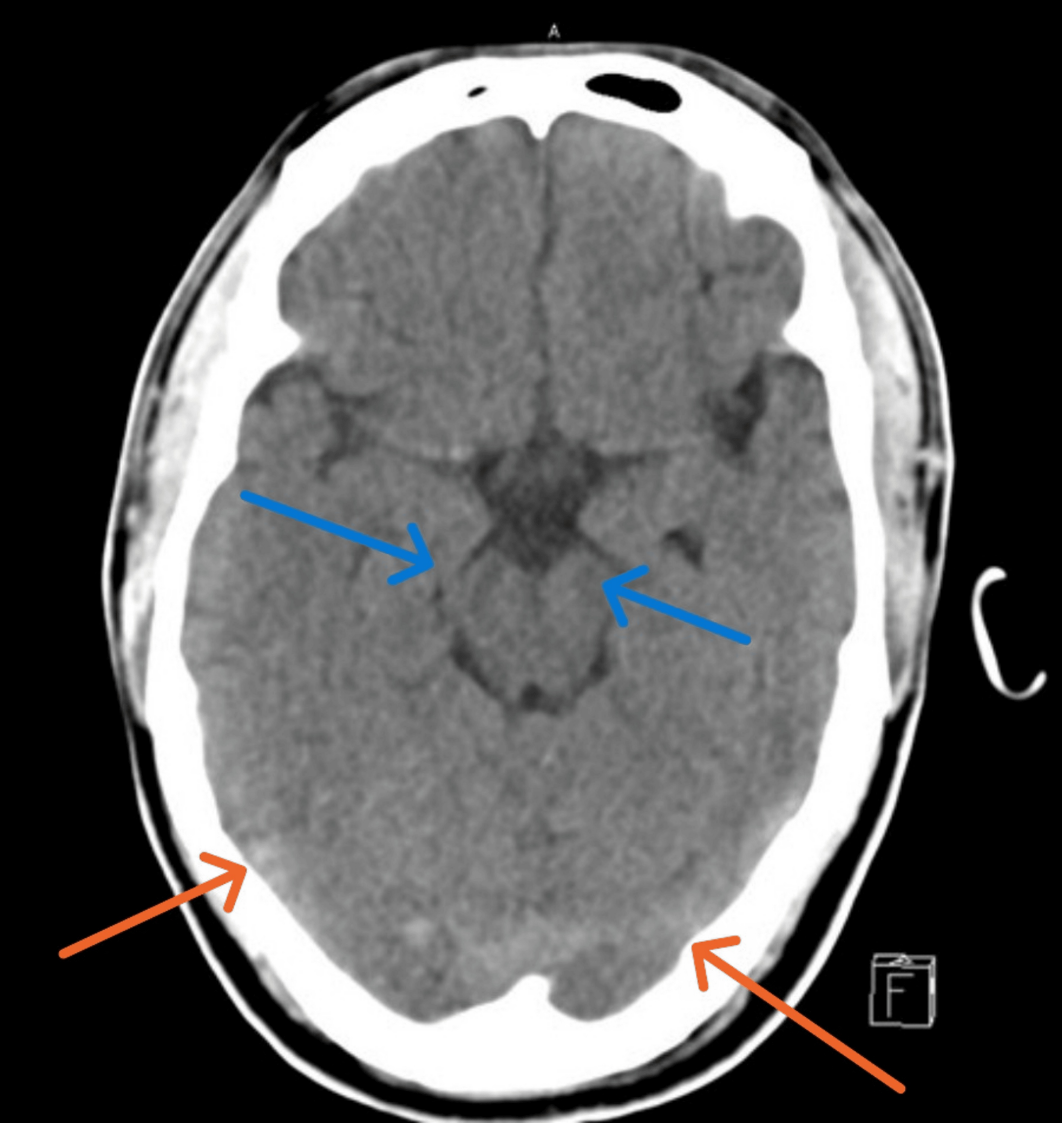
Head CT shows brain stem, thalamus, basal ganglia (blue arrows), and bilateral subcortical hypodensities suggesting ischemic insults (orange arrows).
